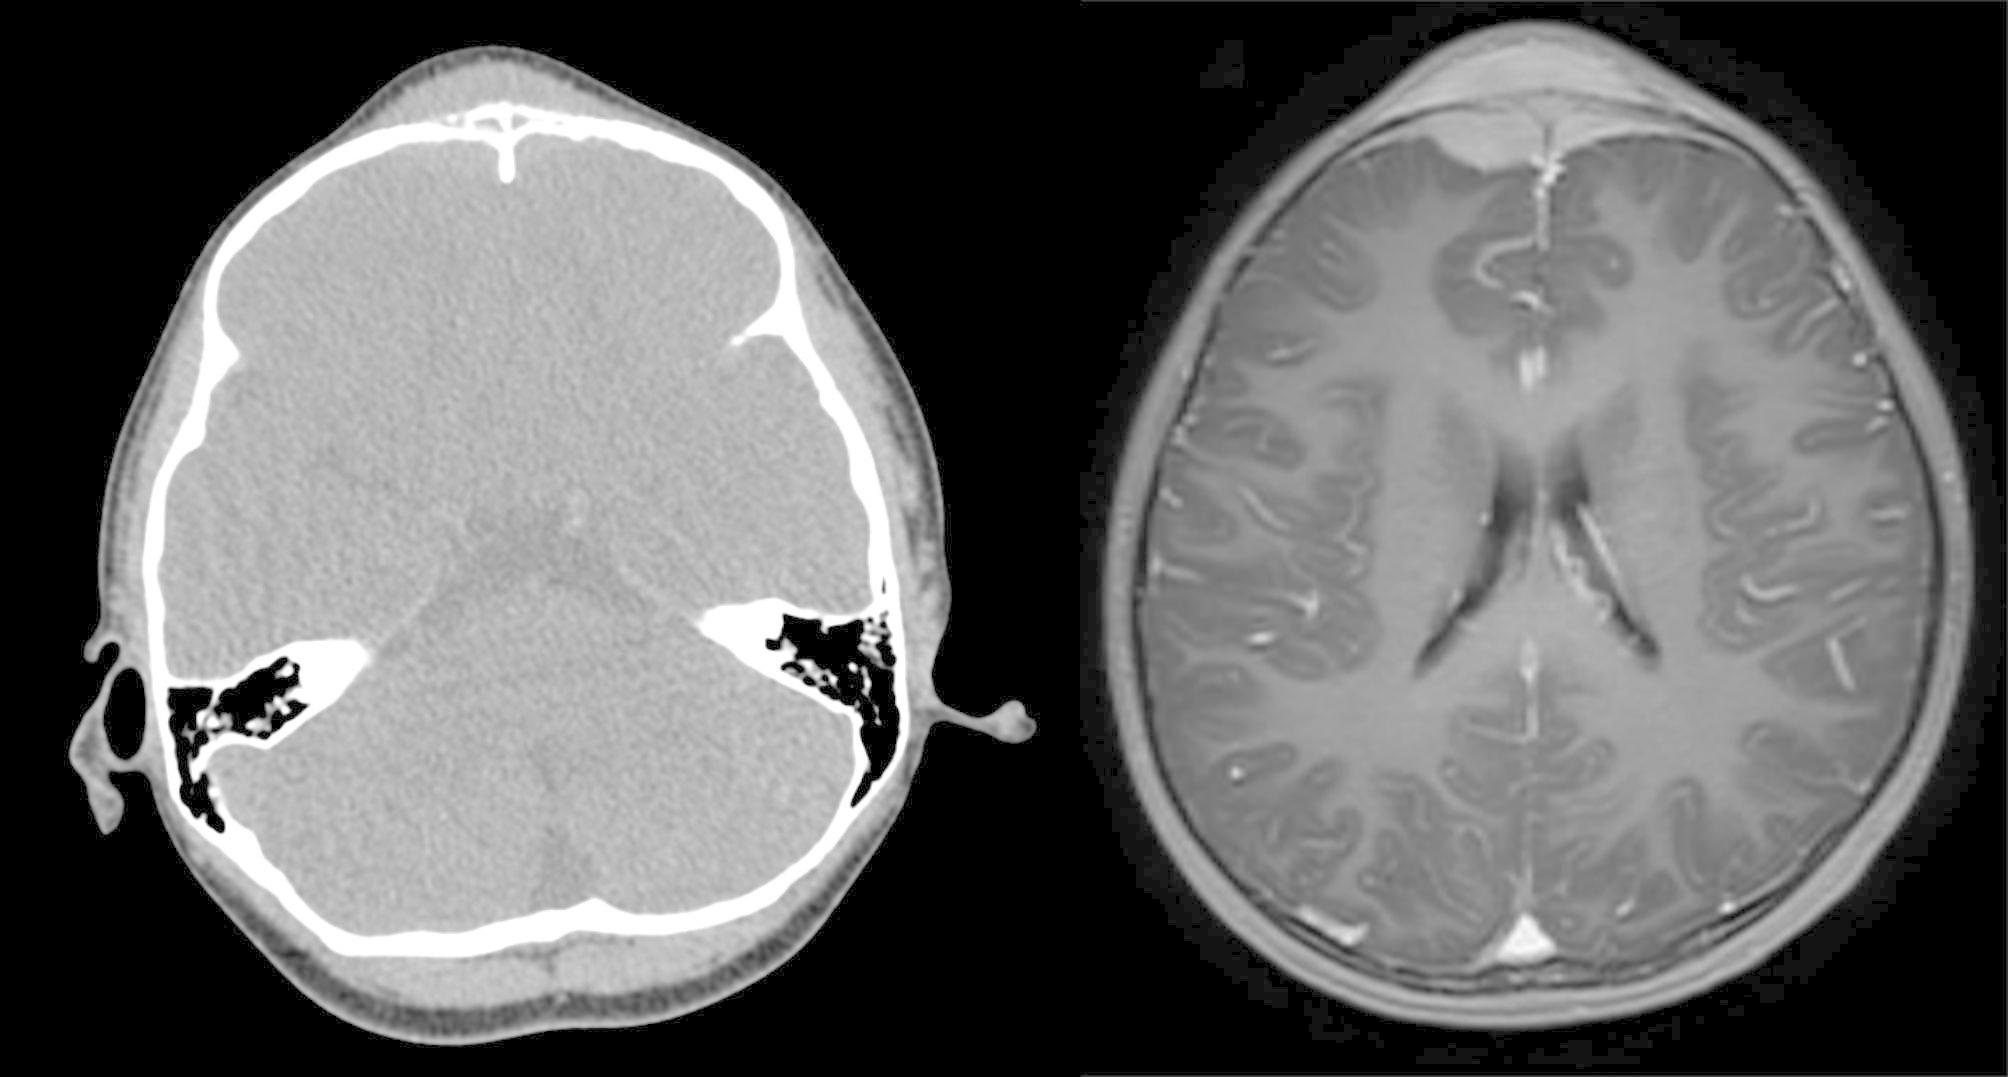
B lymphoblastic lymphoma of the bone, CT and MRI scan. Left image, bone destruction of frontal bone with soft tissue mass. Right image, frontal mass, fusiform mass on inner and outer plates of skull, accompanied by compression of brain tissue
